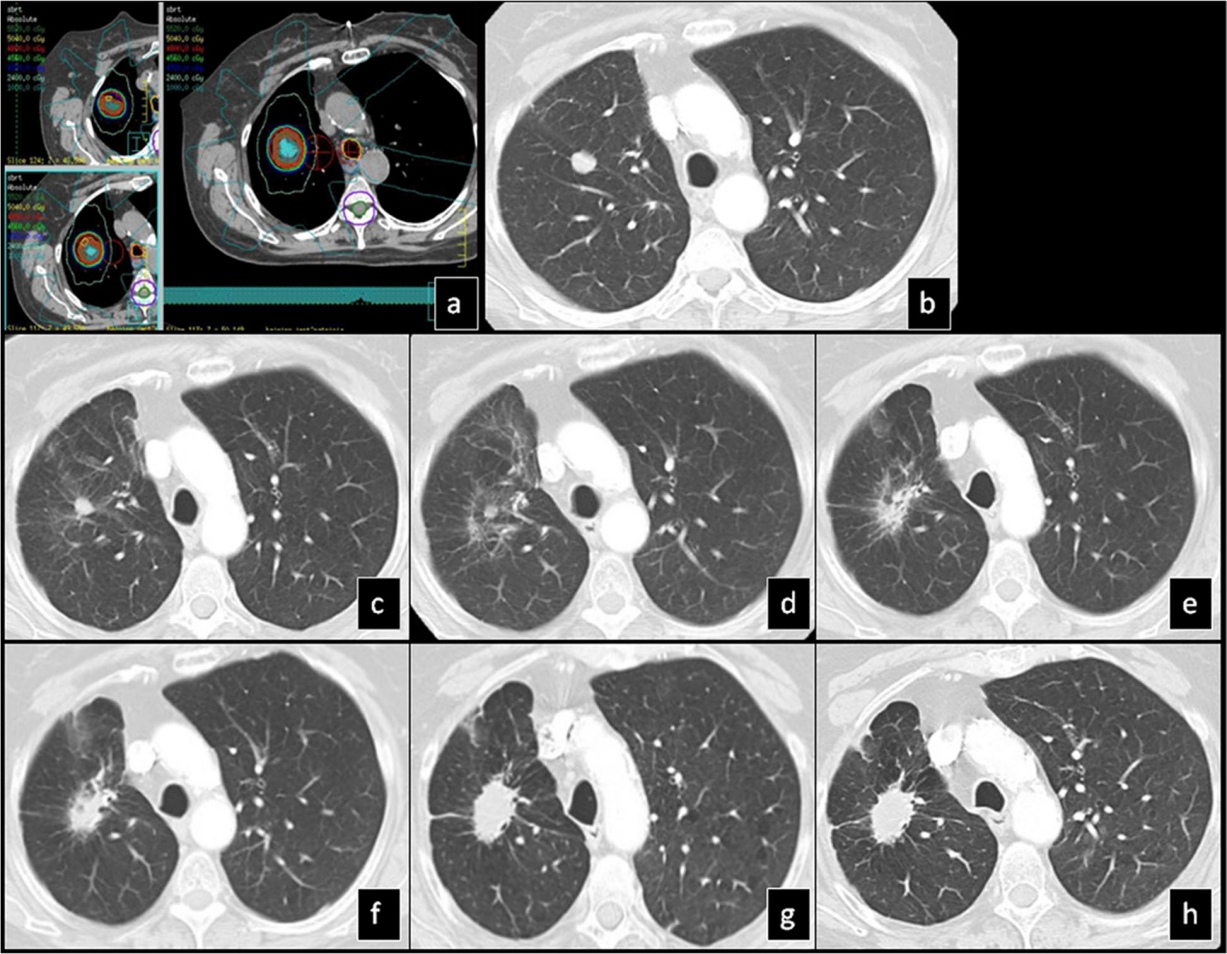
SBRT-treated lung cancer demonstrates stabilization at 61 months: a) Isodose multiplanar images for SBRT planning. b) Pre-SBRT CT shows a solid nodule. c) 10 months (mo) post-SBRT the primary lesion slightly decreased in size with development of diffuse ground glass opacity surrounding the nodule. d) 24 mo post-SBRT “the orbit sign” pattern developed. 40 (e) and 49 mo (f) post-SBRT there was gradual increase in density “filling in” the area between the irradiated nodule and the surrounding curvilinear density gradually obscuring the “orbit sign”. g) 61 mo post-SBRT there was stabilization of “mass-like” pattern. h) 72 mo post-SBRT there was no interval change
